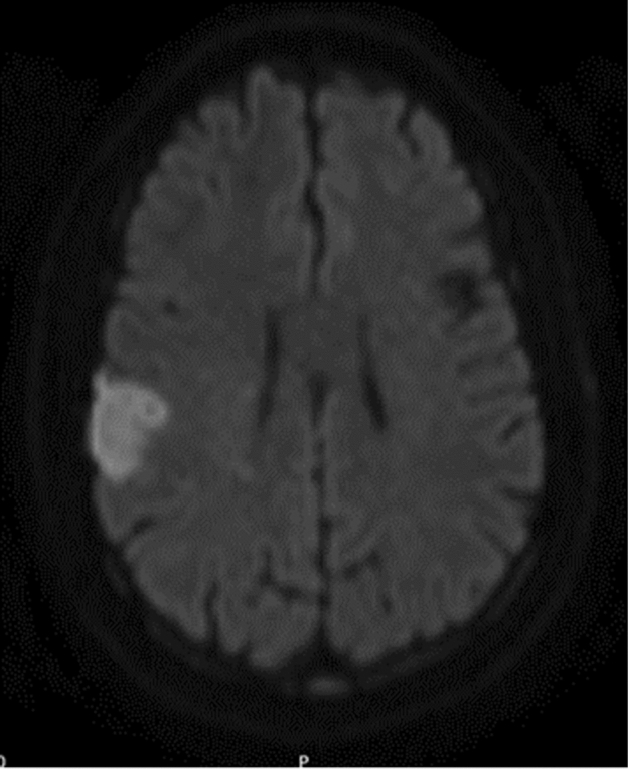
Acute stroke in a patient with paroxysmal nocturnal hemoglobinuria, showing an right hemispheric acute and a left frontal chronic infarct on MR DWI sequence
